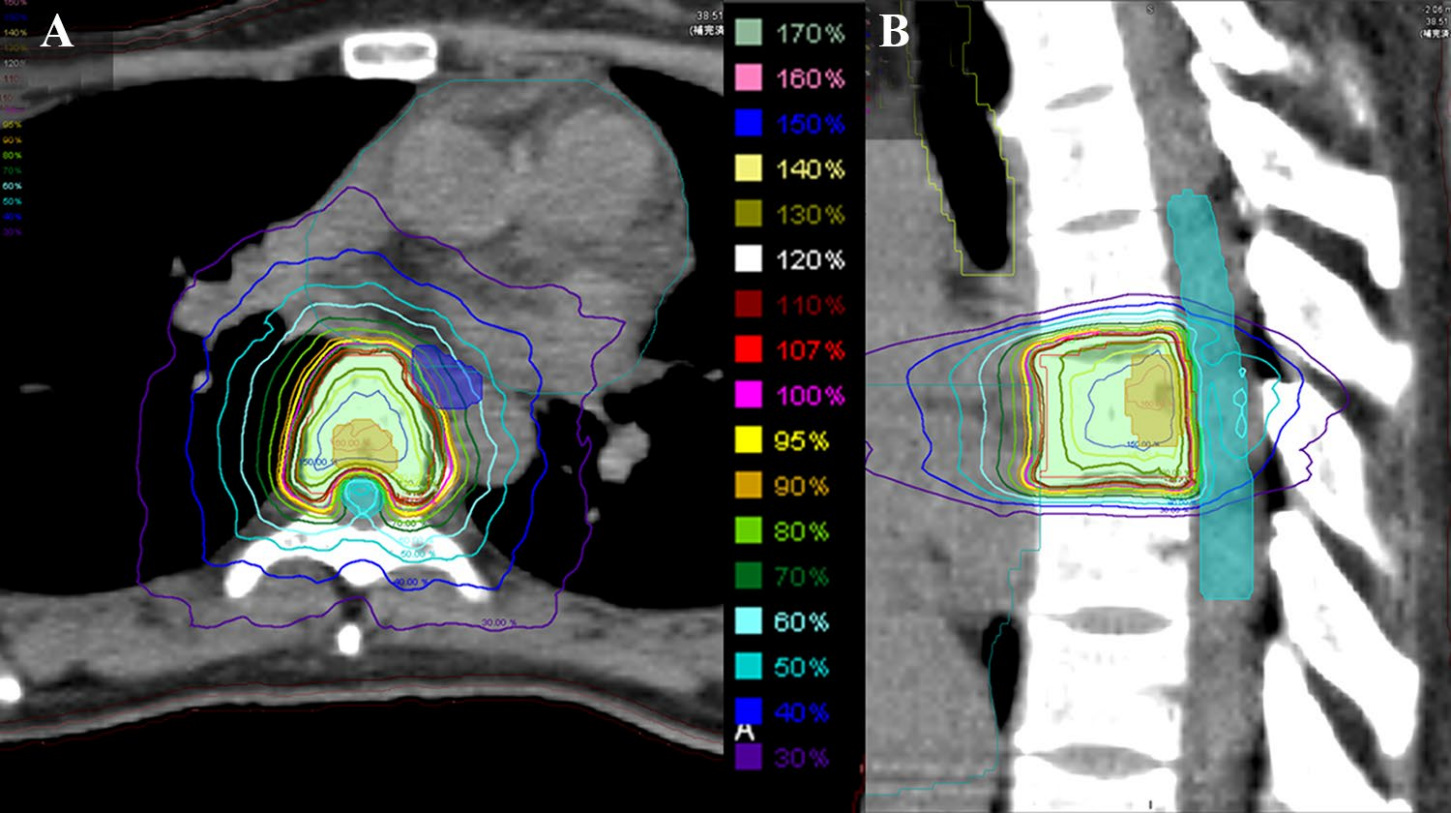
Images of a 33-year-old woman with metastatic T-6 breast cancer. A Axial and B sagittal computed tomography images with dose distribution of stereotactic body radiation therapy
